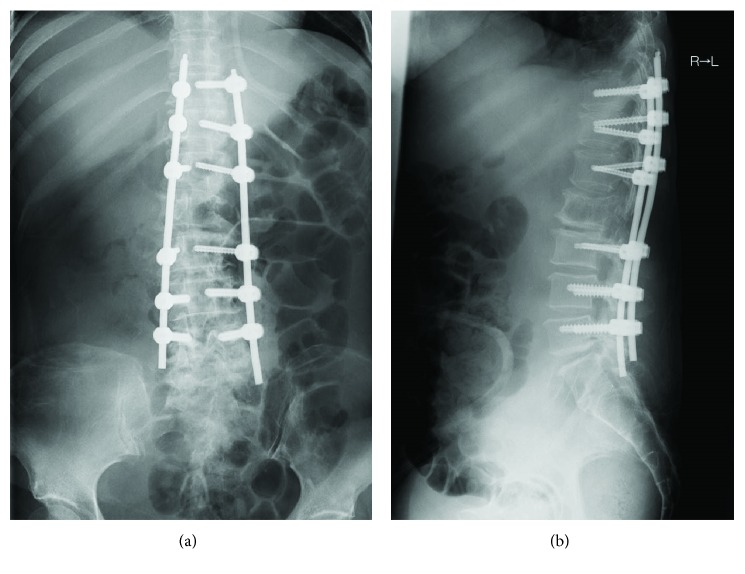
Postoperative radiograph of the anteroposterior view (a) and lateral view (b).
